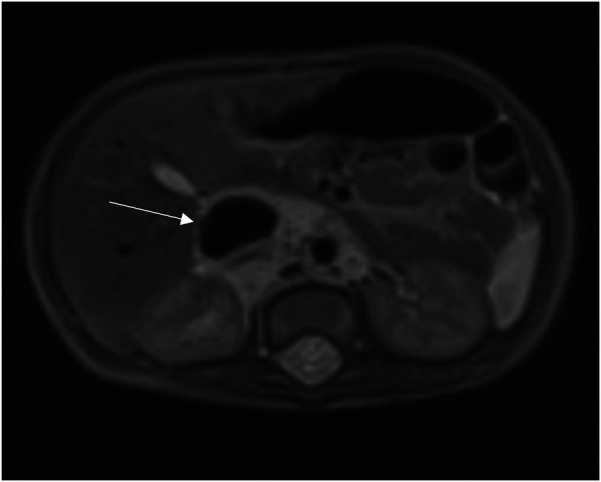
Dilated Inferior Vena Cava (IVC) on axial section at MRI.
